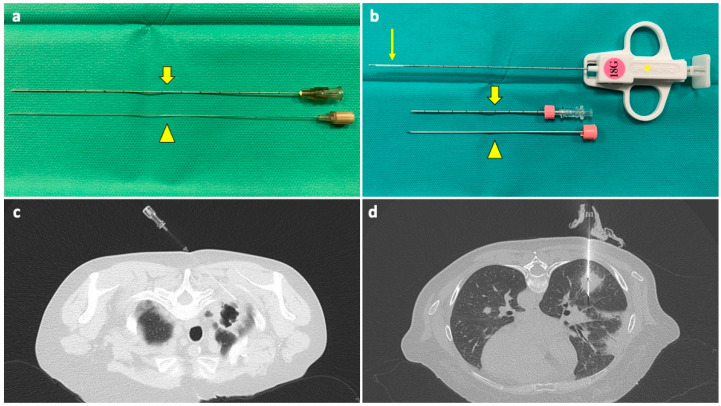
(a) A 22G FNA composed of an outer cannula (thick arrow) and an inner stylet (arrowhead); when in place inside the target lesion, the stylet is removed, and the cannula is connected to a syringe for aspiration. (b) A 18G CNB system composed of a coaxial needle, comprising an outer cannula (thick arrow), an inner stylet (arrowhead), and a biopsy needle (asterisk) with a sample notch at its tip (thin arrow); when the coaxial needle is in place, the stylet is removed, and the biopsy needle is inserted through the cannula. A biopsy gun activates a cutting cannula that moves over the sample notch, cutting the tissue inside it and allowing for collection. (c) The tip of a FNA needle has been positioned under CT guidance into the solid peripheral portion of an excavated lesion in the right upper lobe of a neutropenic 60-year-old woman with a history of breast cancer and a previous negative BAL. (d) The sample notch of a CNB needle is positioned under CT guidance into a solid mass in the lower right lobe of a 53-year-old neutropenic man with multifocal pneumonia, not responding to medical therapy, and with previous negative BAL. Abbreviations—BAL: bronchoalveolar lavage; CNB: core needle biopsy; CT: computed tomography; G: gauge.
